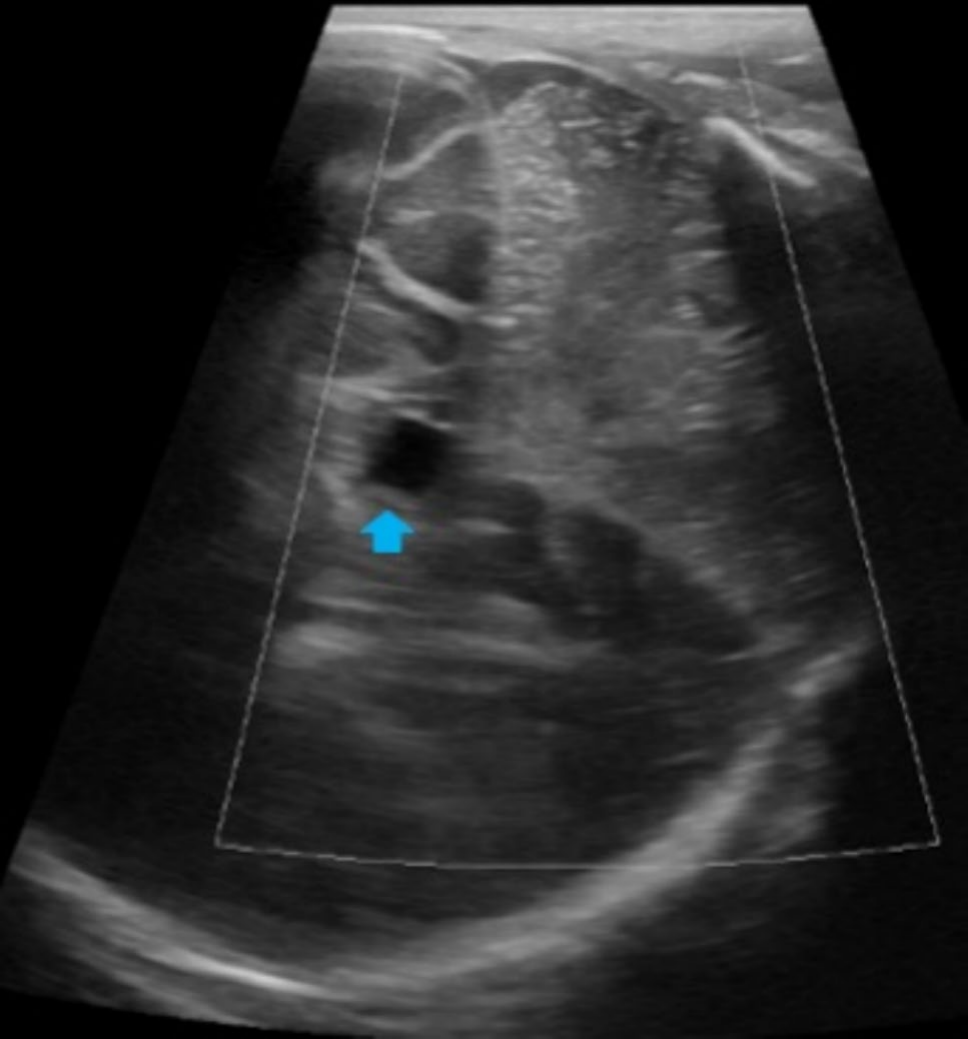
Cerebral ultrasound of 2-day-old full term male showed cavum veli interpositi, appearing as an interhemispheric well-defined unilocular anechoic cyst on mastoid view (blue arrow)
